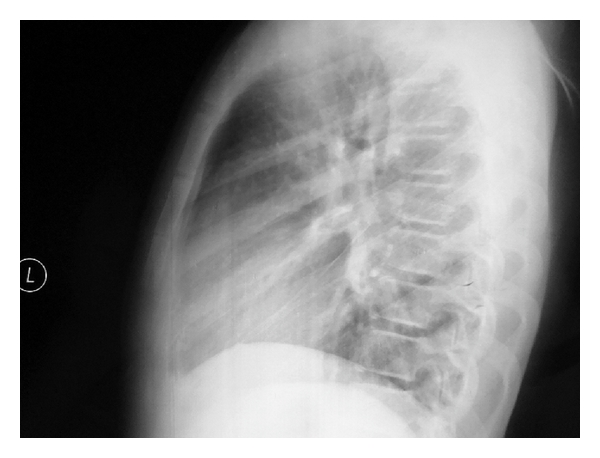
Lateral chest-X-ray after 6 months of reduction. There is a callus-like formation around the dislocated and deducted part of the sternum.
